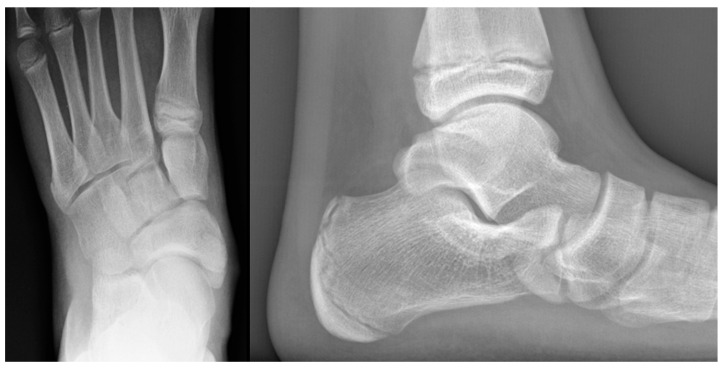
Radiograph of a 10-year-old male patient with flatfoot and a prominent accessory navicular bone.
